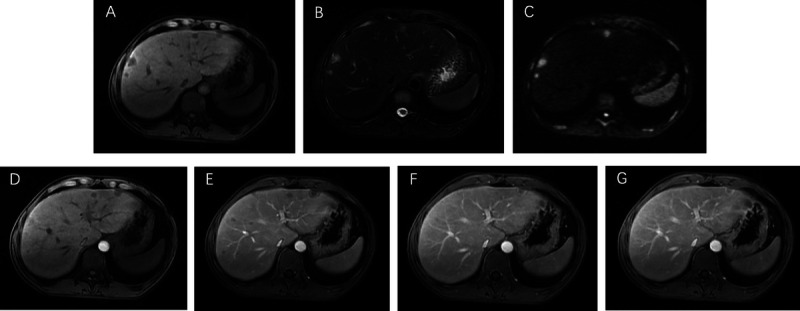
Abdominal MRI revealed multiple intrahepatic lesions: hypointense on T1WI (A) and hyperintense on T2WI (B). DWI (C) showed restricted diffusion with marked hyperintensity. Contrast-enhanced imaging revealed peripheral ring enhancement in the arterial phase (D), centripetal enhancement during the portal venous (E) and delayed phases (F), and prominent enhancement in the late delayed phase (G).
